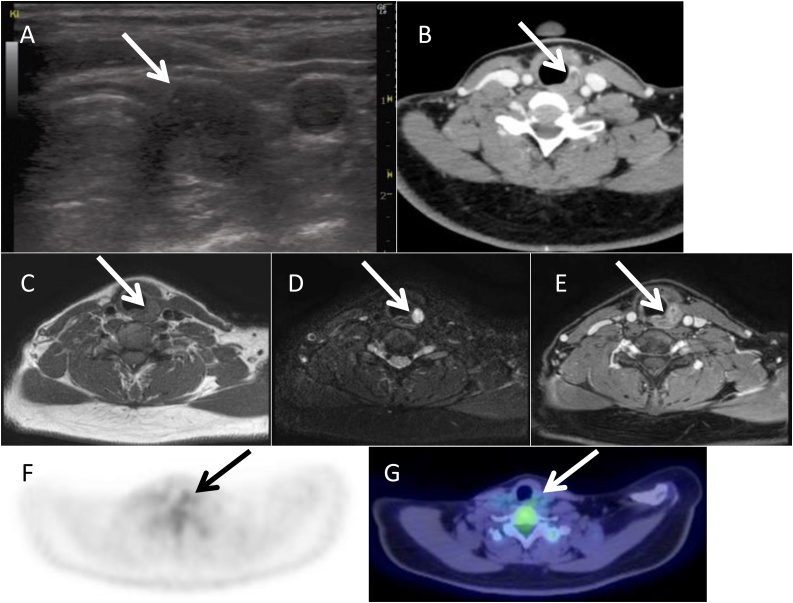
Preoperative image. (A) Ultrasound showed the 15 mm neck mass in the left thyroid bed. (B) A contrast enhanced CT scan of the neck reveal a tumor in the enhanced normal thyroid tissue. (C–E) Axial T1 weighted images demonstrated isosignal intensity mass, which attached to trachea and esophagus. The recurrent tumor was distributed a high signal lesion Axial T2 weighted MR images, and contrast enhanced T1 weighted images clearly showed that tumor did not invade trachea, esophagus, and neck great vessels. (F, G) ^18^F-FDG slightly accumulated to the recurrent tumor site in the positron emission tomography.
